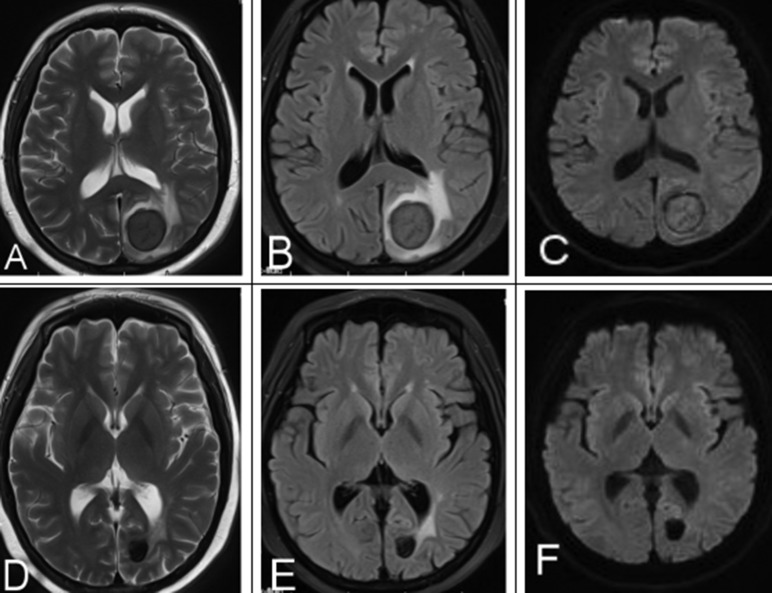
A 25-year-old female with a history of AML underwent chemotherapy and MRI follow-up (A–C) MRI signal intensity was obvious and lower than pre-treatment on T2WI, FLAIR, DWI, indicating a good treatment response. (D–F) Post-treatment mass on T2WI, FLAIR, DWI.
